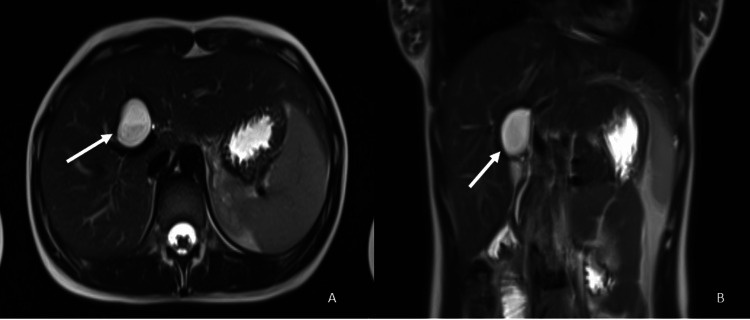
Preoperative contrast-enhanced upper abdomen MRI: (A) T-2 weighted sequence axial view, (B) coronal view. The arrow represents the cystic lesion in IV A segment of the liver.
